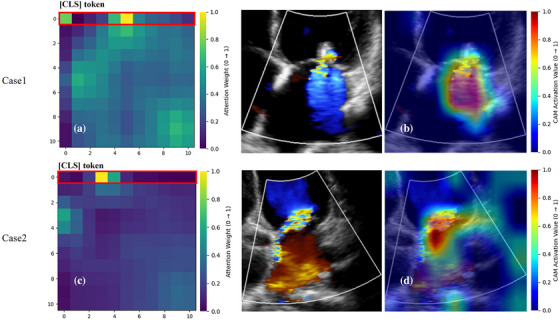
Activation maps of the attention‐based central‐eccentric MR classification model (Case1‐central MR and Case2‐Eccentric MR). (a) and (c) Attention matrices across 11 input frames (frame 0 is [CLS] token) for two representative cases: The highlighted 0th row (top row, red box) indicates the attention weights assigned by the [CLS] token to each frame. Warmer colors (yellow—red) denote higher attention scores (see color bar on the right), while cooler tones (blue—green) indicate lower attention. In both cases, the model predominantly attends to the frame with maximal regurgitant jet intensity—consistent with clinically relevant timepoints. (b) and (d) Left: Original color Doppler echocardiographic images of the maximum regurgitation frame. Right: Corresponding attention heatmaps overlaid on the same frames show that the model can pay attention to the shape of MR in the left atrial surface.
